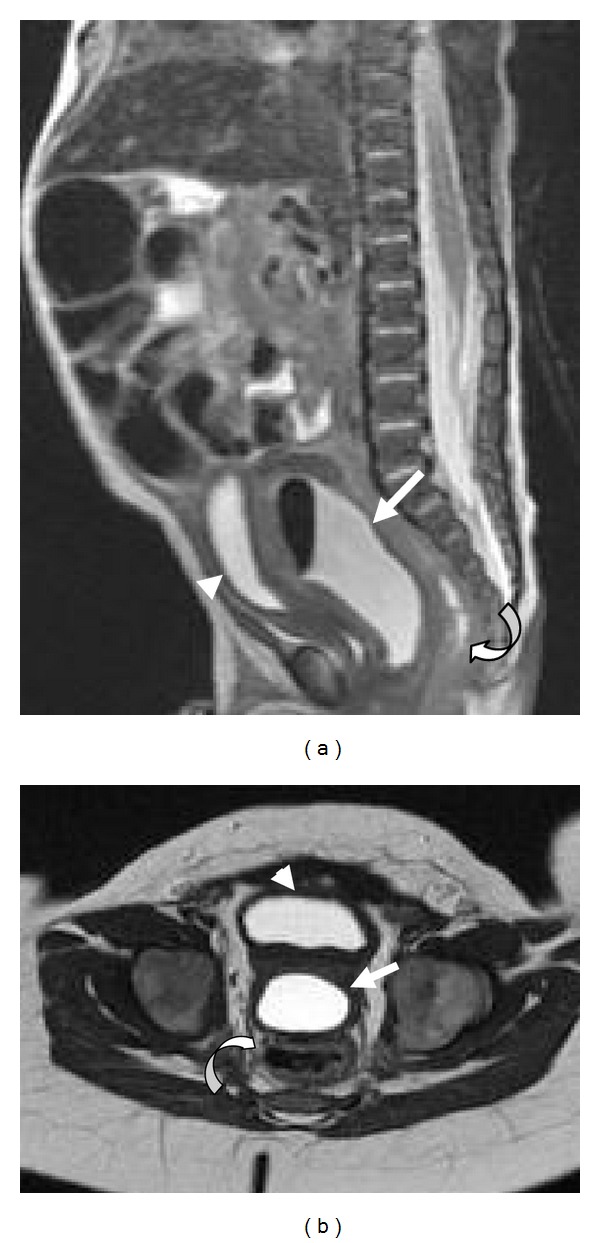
Sagittal (a) and axial (b) T2 turbo spin echo (TSE) MR image show a T2 hyperintense cystic structure representing the prostatic utricle cyst (white arrow), encapsulated by a rim of T2 isointense prostatic tissue, located between the rectum (curved arrow) and urinary bladder (arrowhead).
